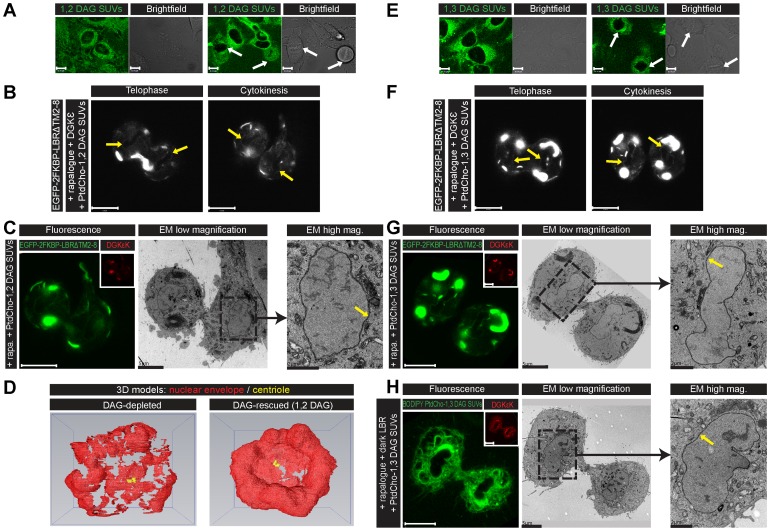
1,2- and 1,3-DAG rescue the fragmented NE phenotype. (A) Confocal images of live HeLa cells 1 min after addition of small unilamellar vesicles (SUVs) containing BODIPY-PtdCho and unsaturated 1,2 DAG (80∶20 mole% respectively). Incorporation of SUVs (green) into interphase and metaphase (white arrows) cells are shown. (B) LBR localisation during mitosis in rapalogue-treated, LBR and DGKε-expressing HeLa cells after addition at metaphase of SUVs containing PtdCho and unsaturated 1,2 DAG (80∶20 mole%). NE reformation (yellow arrows) was rescued. (C) Ultrastructure of the NE (yellow arrow) of the same cell at cytokinesis imaged using CLEM (Fig. S4). LBR localisation in green, DGKε in red. Serial sections are shown in Movie S8. (D) Comparison of 3D models reconstructed from serial images of DAG-depleted (left panel) and DAG-rescued (right panel) cells shows the NE reformed in the presence of 1,2 DAG. (E–G) Similar results as in (A–C) respectively were obtained with SUVs of the non-C1 domain-binding DAG isomer 1,3 DAG. (H) CLEM images of a rapalogue-treated, dark LBR and DGKε-expressing HeLa cell fixed at cytokinesis, after addition of (60∶40 mole %) SUVs with BODIPY-PtdCho and unsaturated 1,3 DAG. Incorporation of the SUVs into cell membranes in green, DGKε in red. EM images show 1,3 DAG completely rescued NE reformation. Images representative of n = 11 experiments. Scale bars: confocal 10 μm, CLEM as indicated on the images.
